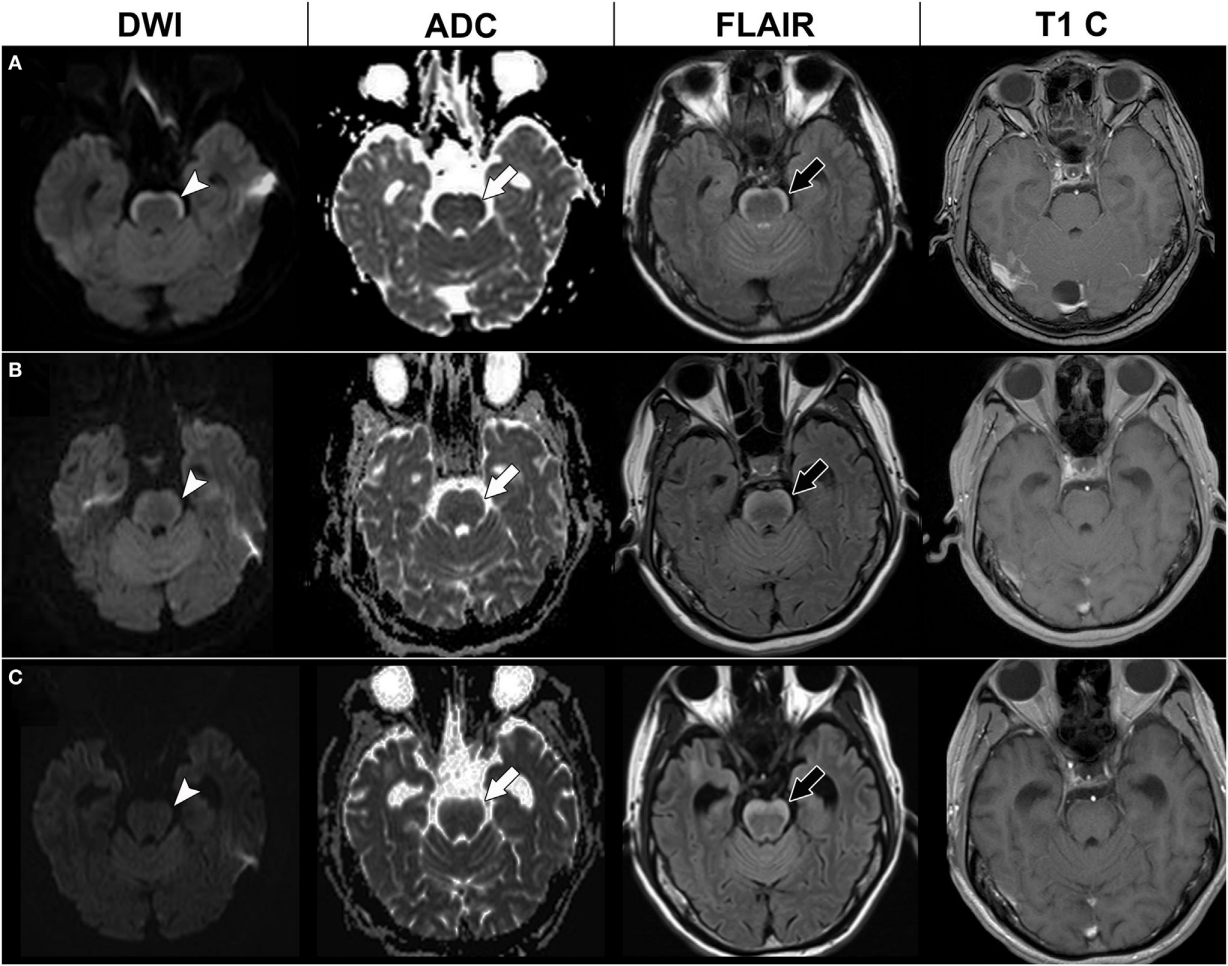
Serial brain magnetic resonance imaging (MRI) of case 1. Initial brain MRI (A) showed a high signal in diffusion-weighted imaging (DWI) at the anterior pons (arrow head) with a low signal in apparent diffusion coefficient (ADC) mapping (white arrow), hyperintensities in fluid attenuation inversion recovery (FLAIR) imaging (black arrow), and no enhancement in T1-weighted imaging with contrast (T1 C). Brain MRI 4 months later (B) and 7 months later (C) showed a thickening of the anterior pons lesion in the FLAIR imaging (black arrow), but gradual resolution of the restricted diffusion pattern in DWI (arrow head) and ADC mapping (white arrow).
